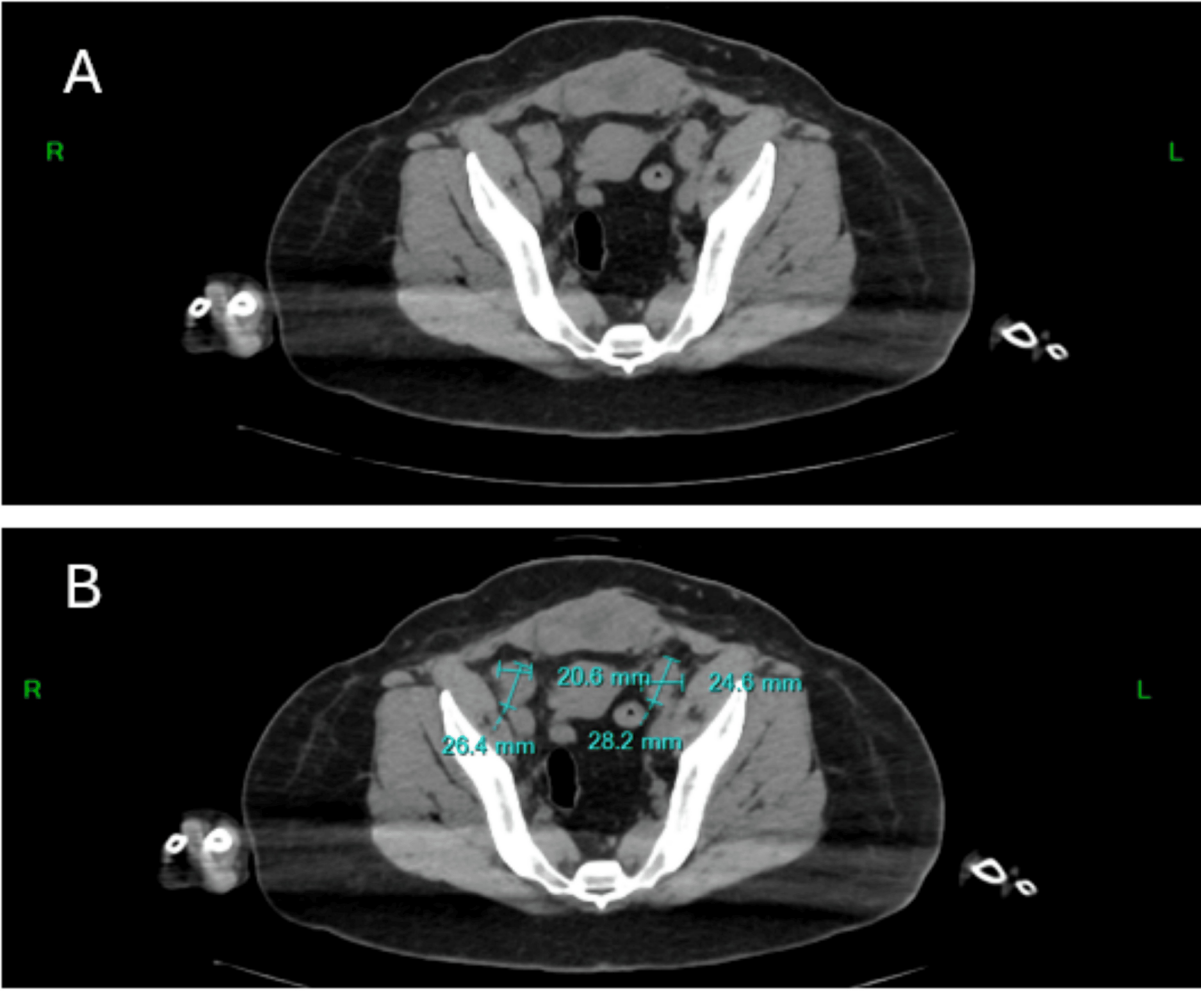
CT abdomen and pelvis. Axial slice of the patient showing the identified masses within the pelvis. (A) The patient's uterus is shown in the top portion of the scan with two masses on either side of it. (B) The measurements are superimposed for identification of the masses on either side of the uterus.
